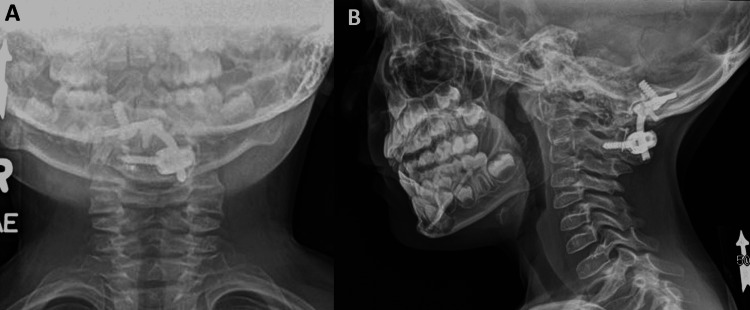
AP and lateral radiographs demonstrating occipital to C2 instrumentation with a unilateral occipital plate and unilateral C2 translaminar screw placement. AP: Anterior to posterior
